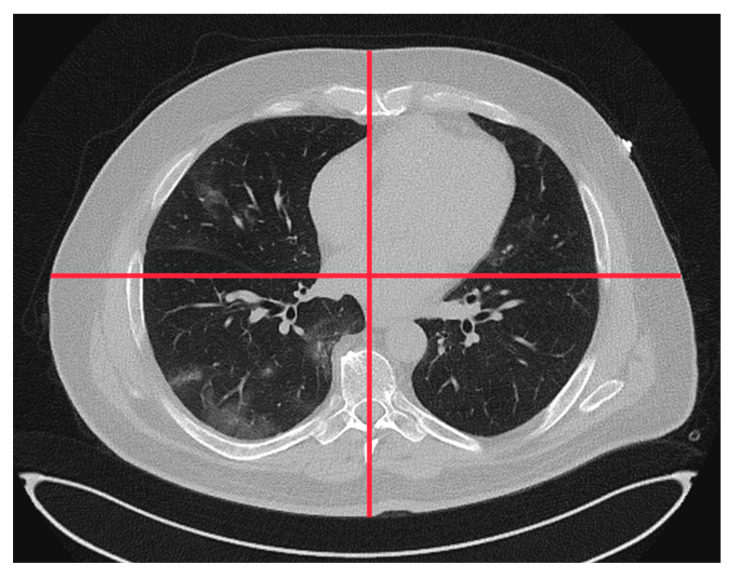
Central anterior–posterior and lateral diameter measured on the CT images (lung window) at the height of the heart.
